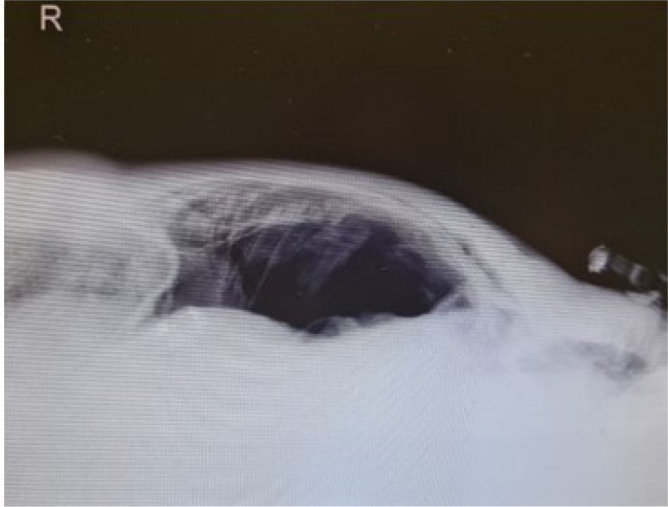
Tangencial X-ray of abdominal showing free gas in abdominal cavity.
